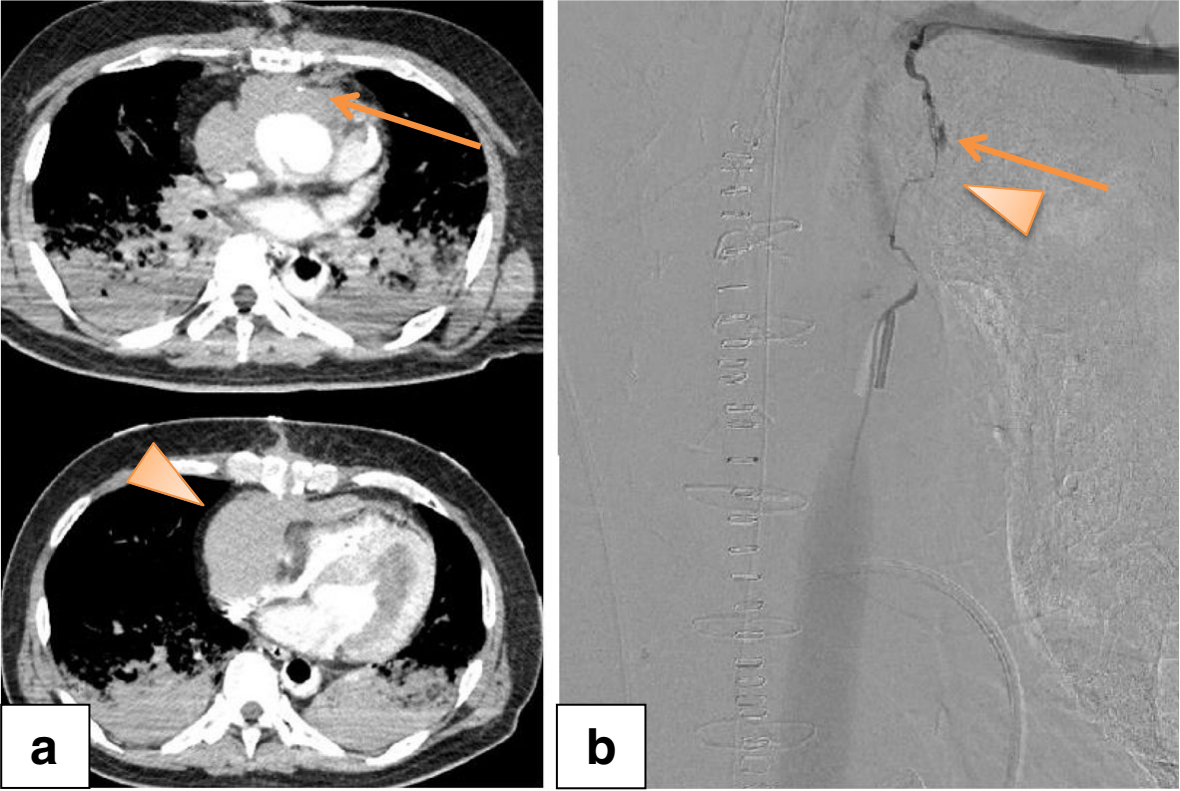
Computed tomography and angiography findings from Patient 3. a Contrast-enhanced computed tomography after the percutaneous coronary intervention revealed contrast extravasation (*arrow*), a hematoma at the mediastinum (*arrowhead*), and compression of the right atrium and ventricle. b Angiography after the contrast-enhanced computed tomography revealed contrast extravasation proximal to the left internal mammary artery (*arrow*) with arterial spasm (*arrowhead*)
